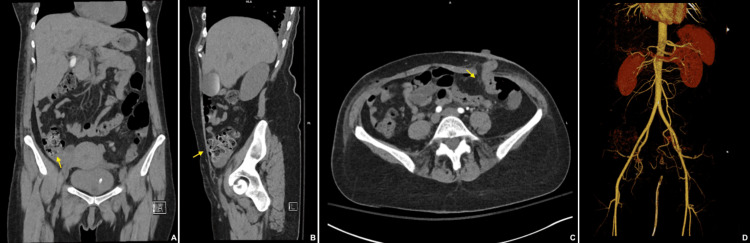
Post-operative computed tomography imaging of the abdomen and pelvis with contrast, demonstrating no evidence of active bleeding, including of the ostomy site (yellow arrows), and visualized small and large bowel. (A) Coronal view. (B) Sagittal view. (C) Transverse view. (D) Angiography.
